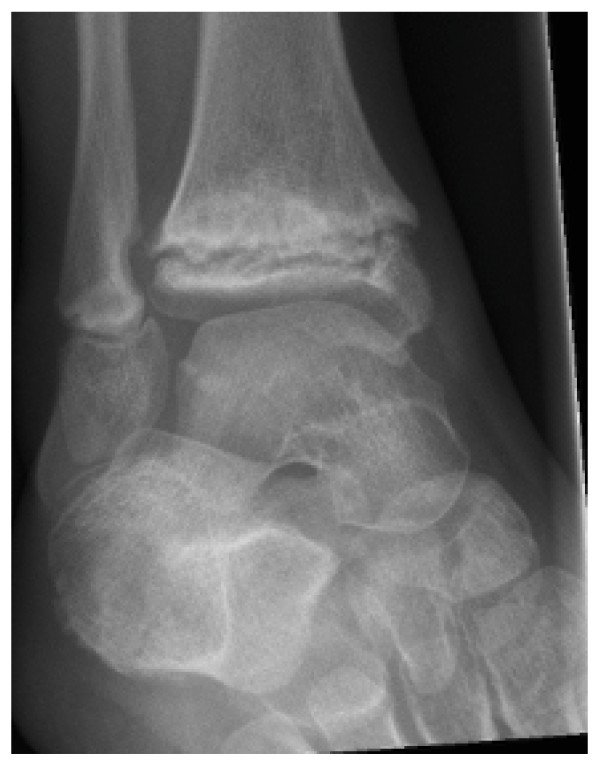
Sibling C - X-ray of right ankle showing abnormal osteolytic process of epiphyses of distal tibia and fibula, aged 8 yo.
